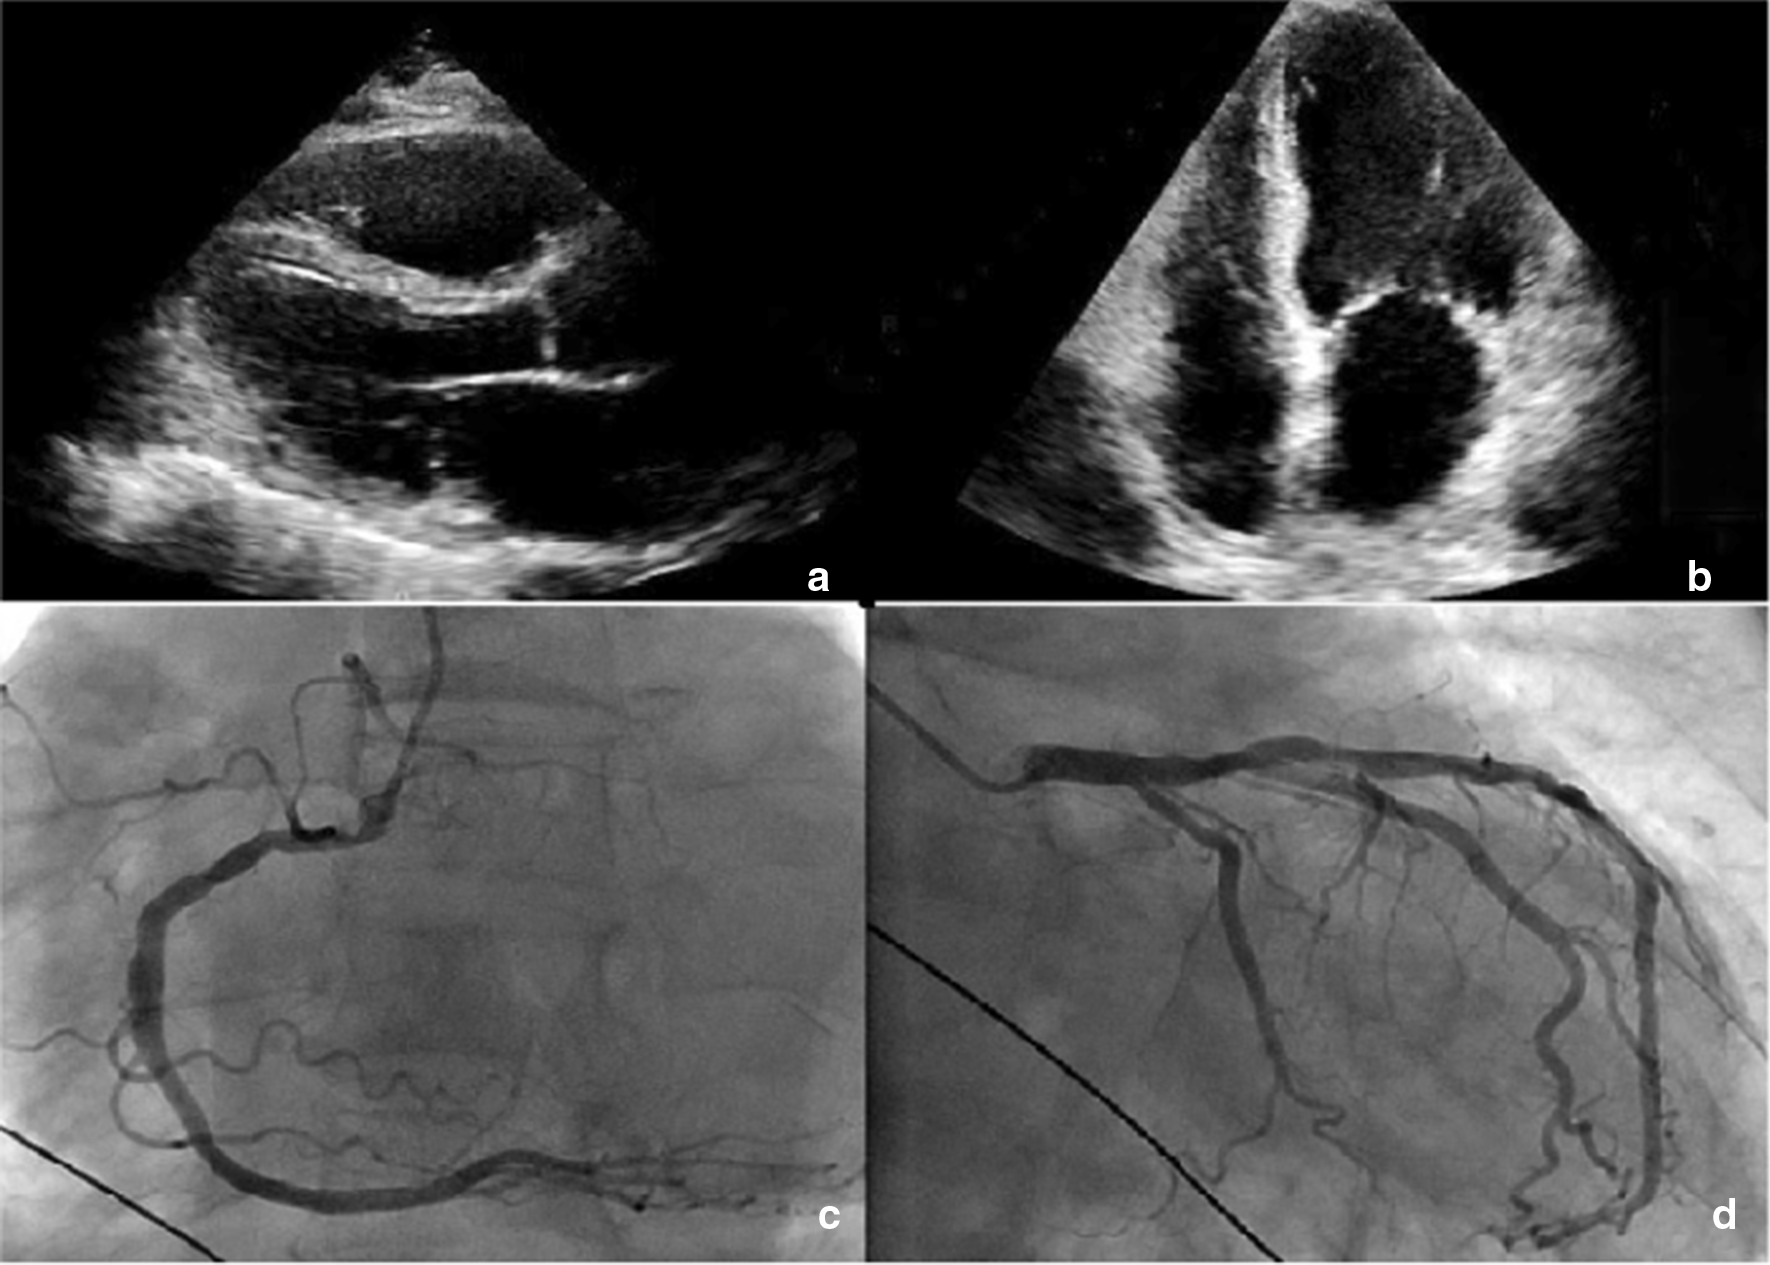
a, b Transthoracic echocardiogram parasternal long axis view and apical 4 chamber view. c, d coronary artery angiography showed non-obstructive mild lesions in the left anterior descending artery and the right coronary artery
